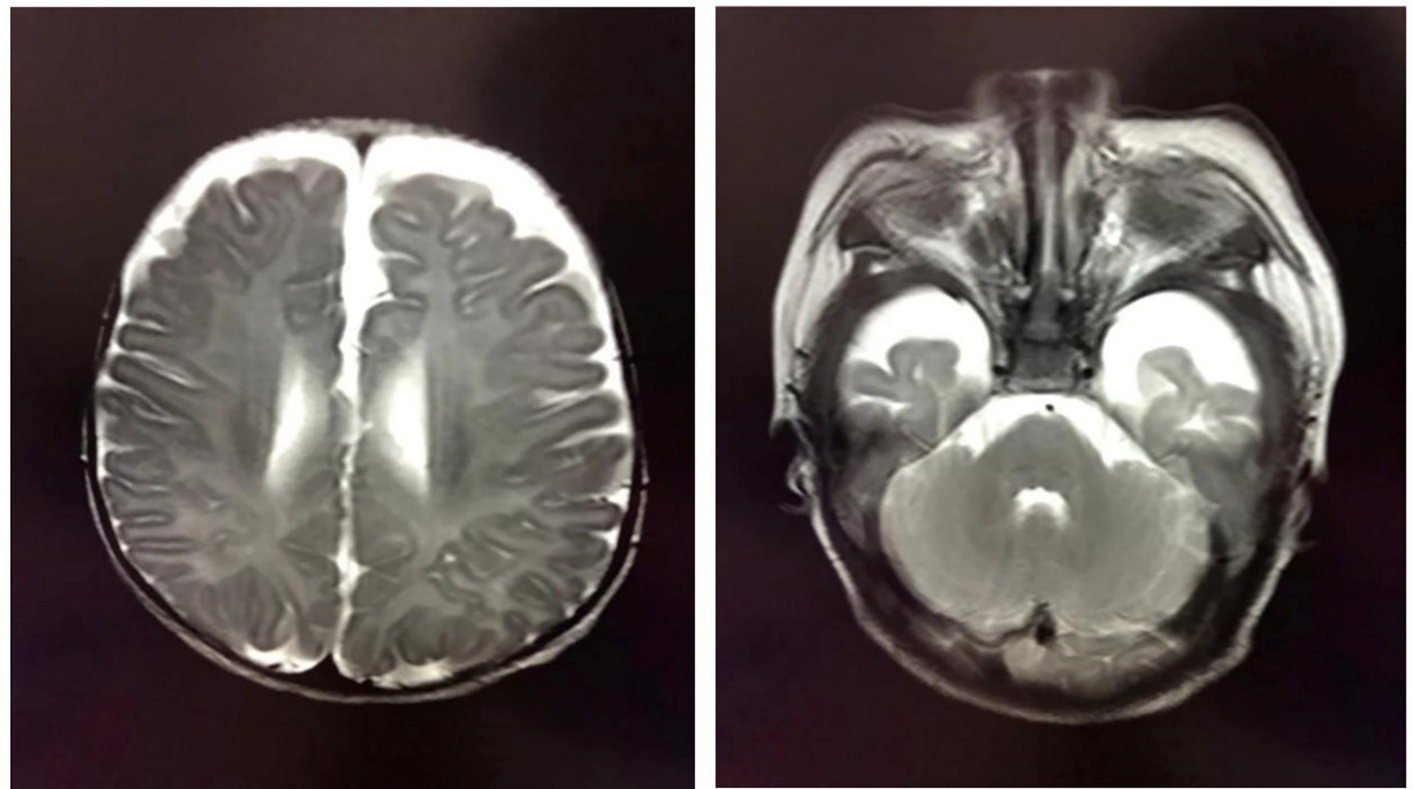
Brain MRI showing the broadened bilateral frontotemporal extracerebral space, which is especially apparent on the left side.
